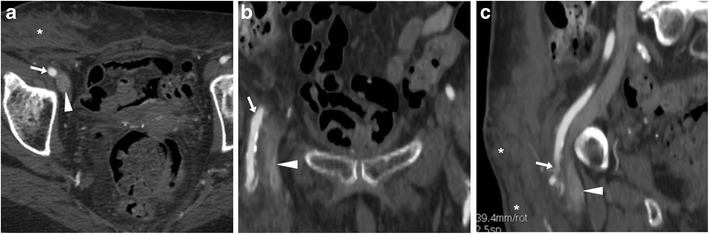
Arterio-venous fistula (AVF) observed 24 h after CA: multiplanar CT-angiographic images showed subcutaneous haematoma (*) and simultaneous opacification of the CFA (arrows) and adjacent femoral vein (arrowheads) consistent with AVF
